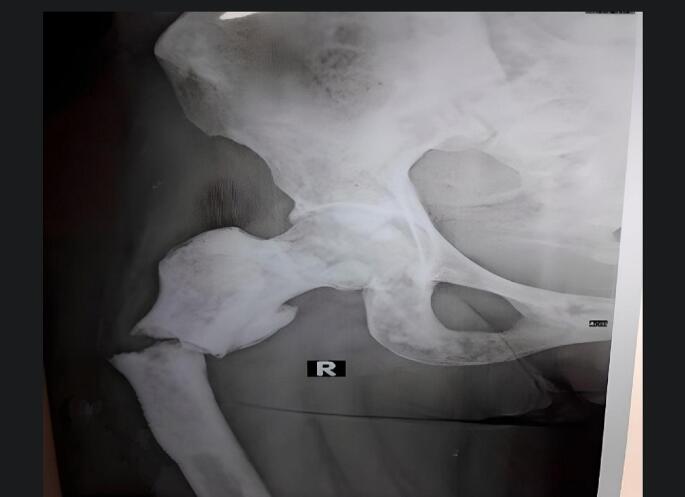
Femur X-Ray shows right transverse subtrochanteric fracture.
